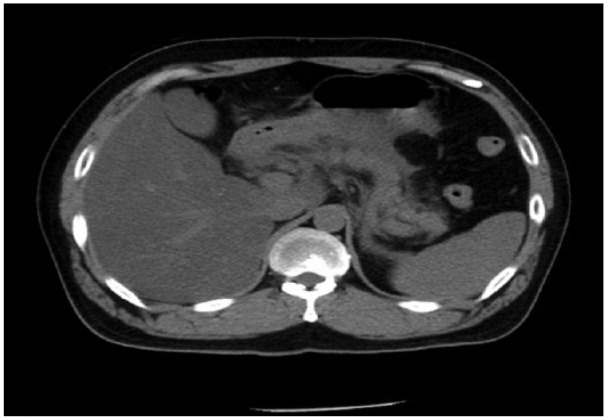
Computed tomography scan showing highly enlarged pancreatic parenchyma with peripheral fluid accumulation, bilateral pleural effusion, and right lower lobe atelectasis. Findings were consistent with acute pancreatitis.
